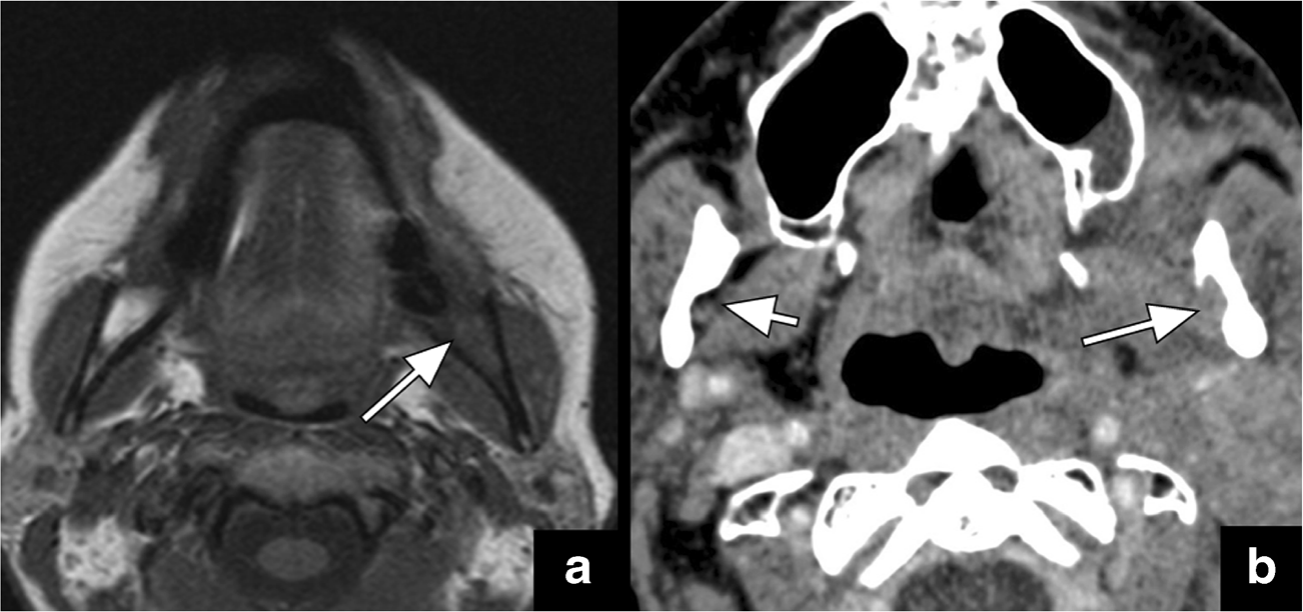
Axial T1-weighted image (a) shows obliteration of the fatty bone marrow in the left mandible (*arrow* in a). The axial CT image (b) of the same patient reveals obliteration of the fat pad at the mandibular foramen (*long arrow* in b), compared to the normal fat pad on the right (*short arrow* in b). These signs are indicative of PTNS along V3 in this patient with history of gingival cancer and V3 numbness
